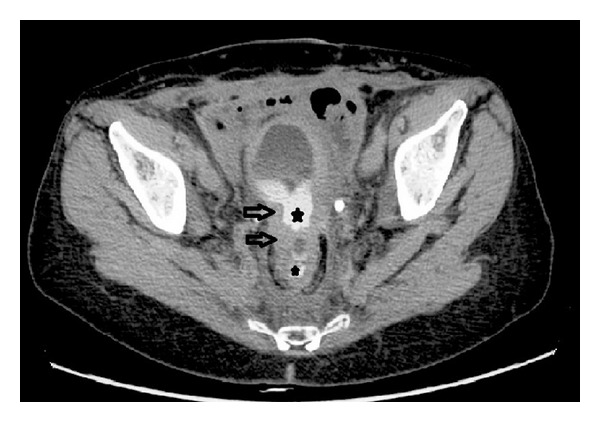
Colovesical fistula: axial image in the delayed phase of CT urogram demonstrates bladder and rectal wall thickening (arrows) with contrast present in both (∗).
